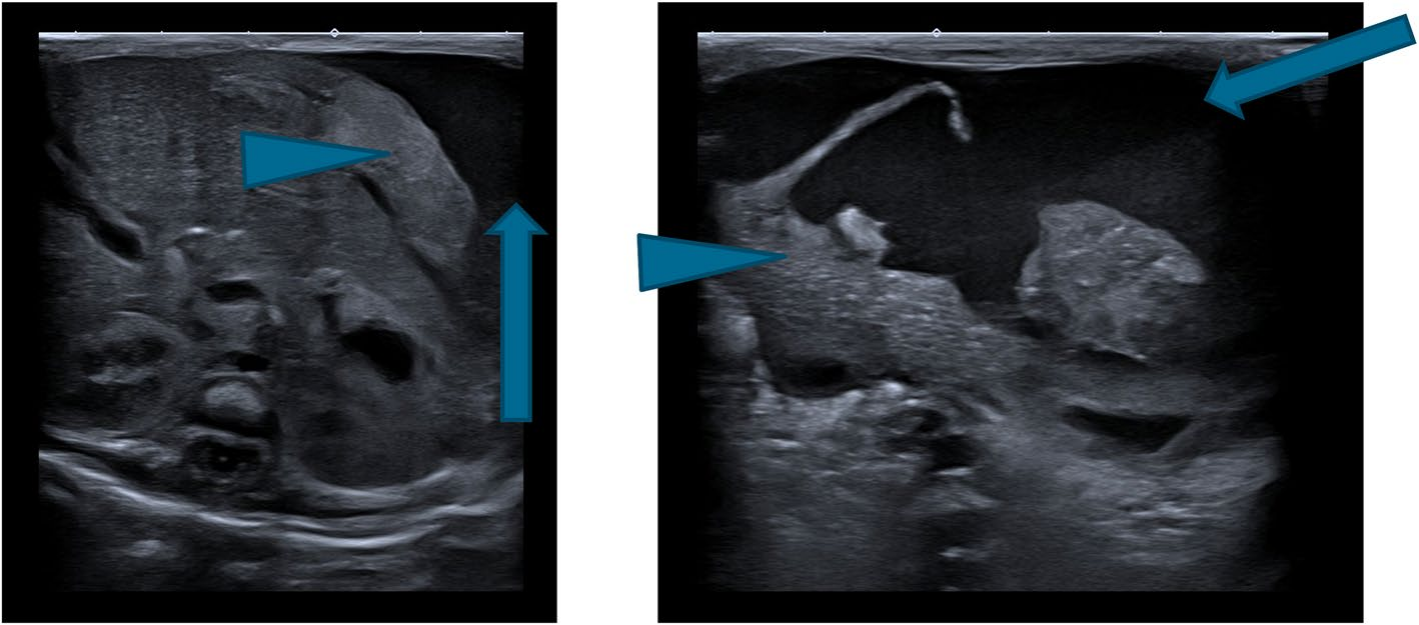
A. Transversal and B. sagittal ultrasound of case 2. The arrow indicates the fluid (hemorrhage) in the left upper abdomen. The arrowhead indicates the splenic parenchyma forming a border of the hemorrhage
